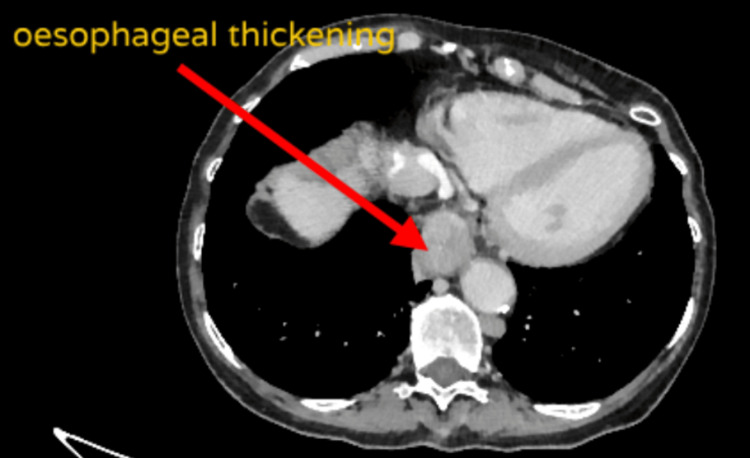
CT-TAP showing concentric esophageal thickening CT-TAP: computerized tomography scan of the thorax, abdomen, and pelvis
